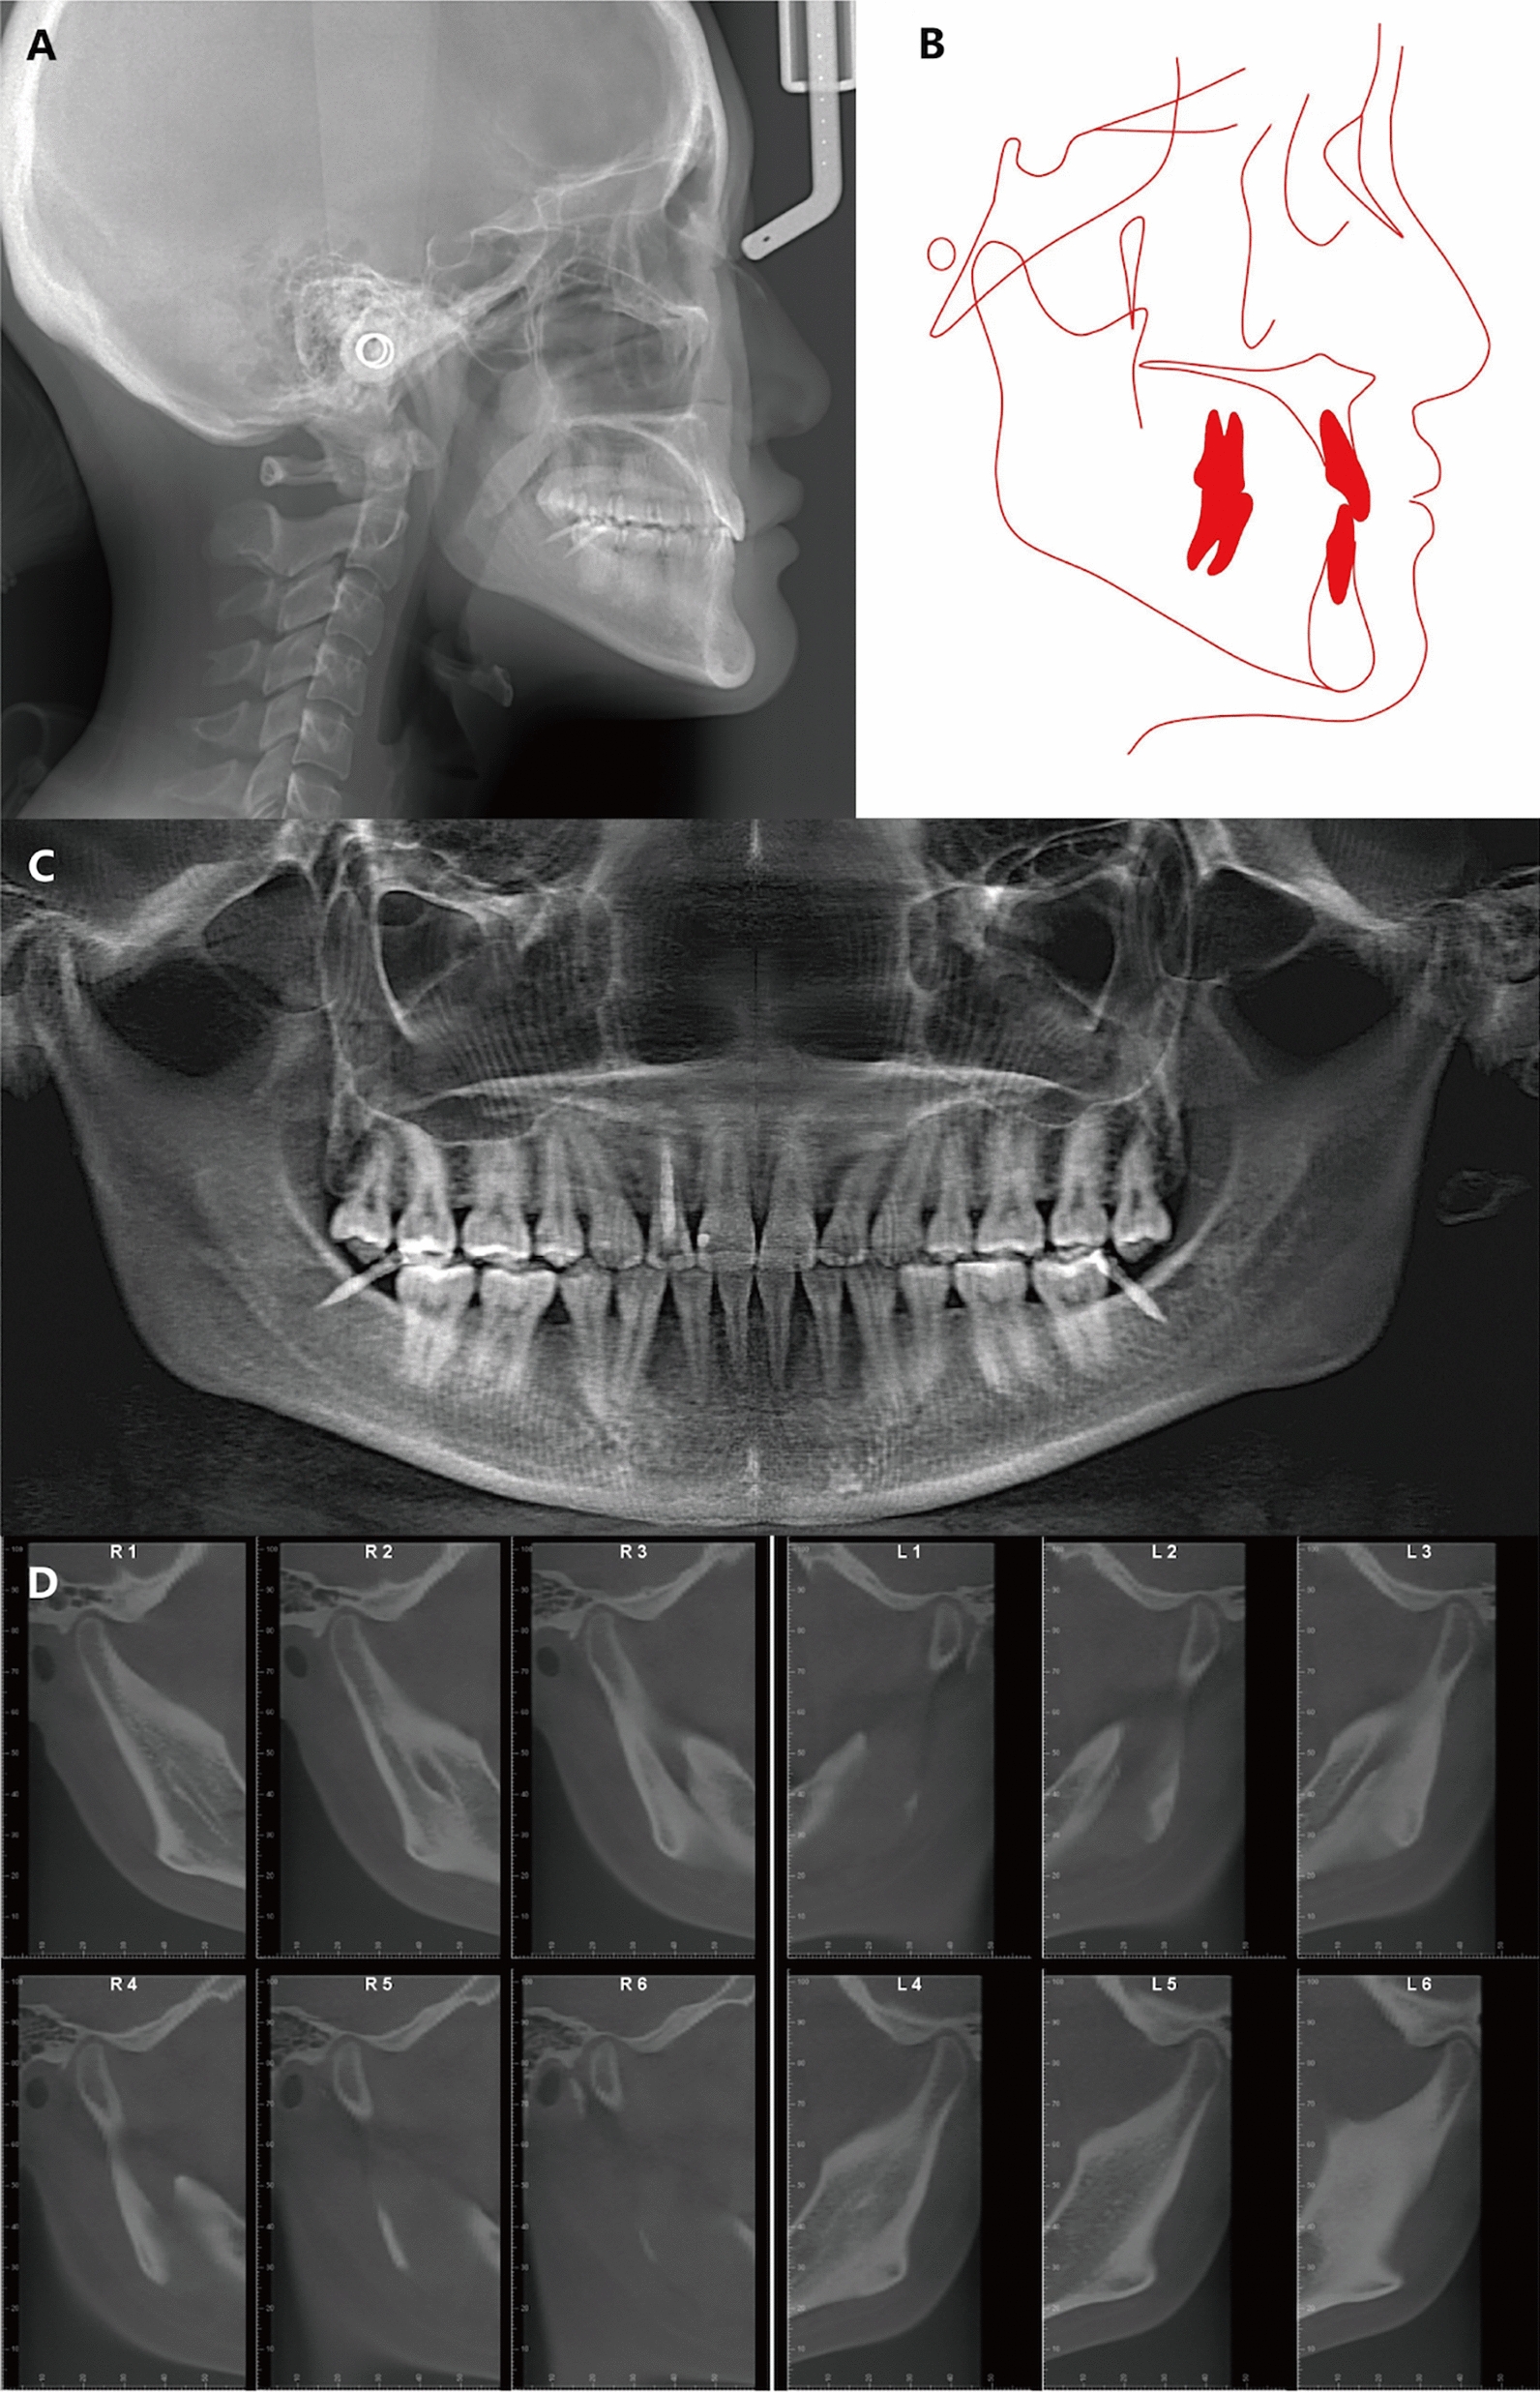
Posttreatment radiographs: A Lateral cephalogram. B Lateral cephalogram tracing. C Panoramic radiograph. D Cone-beam computed tomography image of both TMJs. L, left TMJ; R, right TMJ
